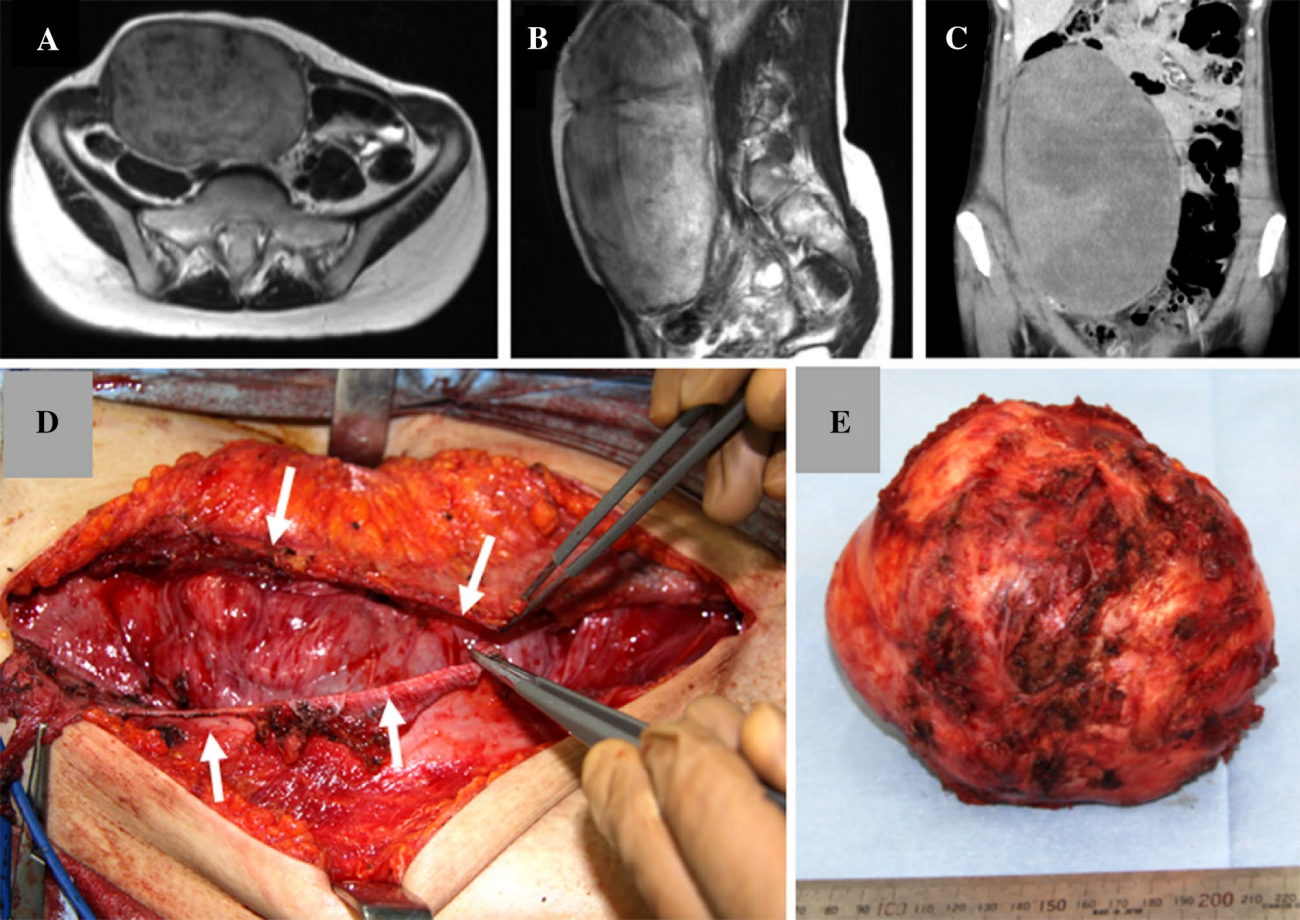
Preoperative images, and findings during fascia-sparing surgery. Patient 1 (A–D). Preoperative MRI and CT. T2-weighted axial plane (A), T2-weighted sagittal plane (B), Contrast-enhanced sagittal plane of CT (C). Fascia (white arrows) was preserved after removal of tumor (D). Patient 4. Removed desmoid detached from fascia (E). (Adobe photoshop CS6 ver.13.0 × 32, Microsoft PowerPoiont 2013).
